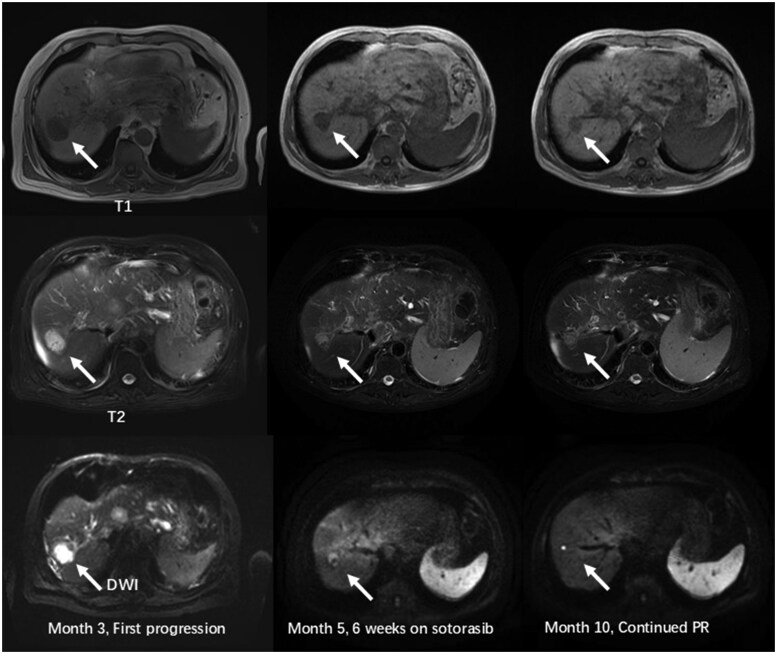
Three-panel T1-weighted magnetic resonance image of the liver. (A) At month 3, first progression showing multiple dark, solid metastatic lesions. (B) At month 5, 6 weeks after starting sotorasib showing those lesions have significantly shrunk and decreased in number. (C) At month 10 showing continued reduction, with only small residual lesions visible.
